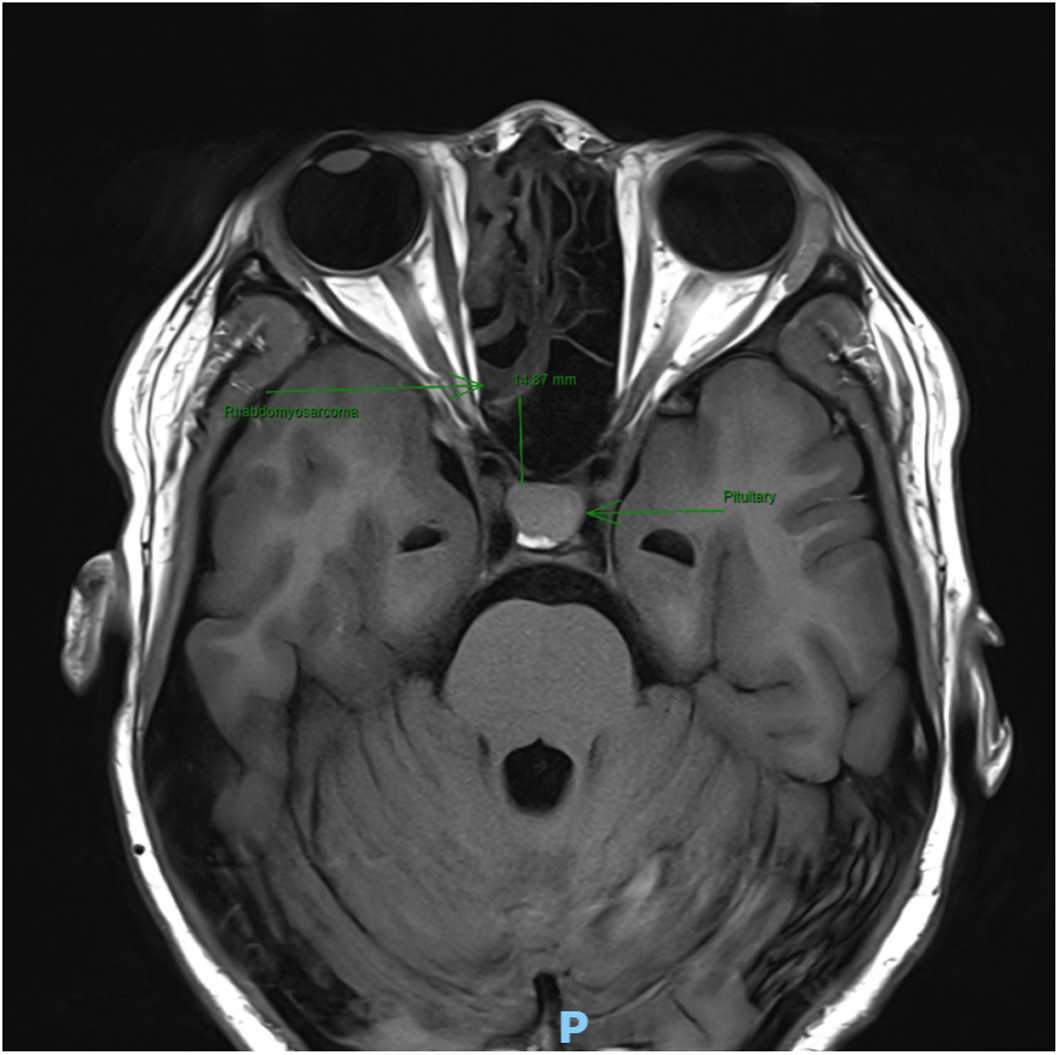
Pre-operative magnetic resonance imaging of the pituitary. The axial T1 weighted magnetic resonance imaging of skull base demonstrates the tumour is radiologically distinct and greater than 10 mm from the pituitary gland. The pituitary bright spot is intact.
